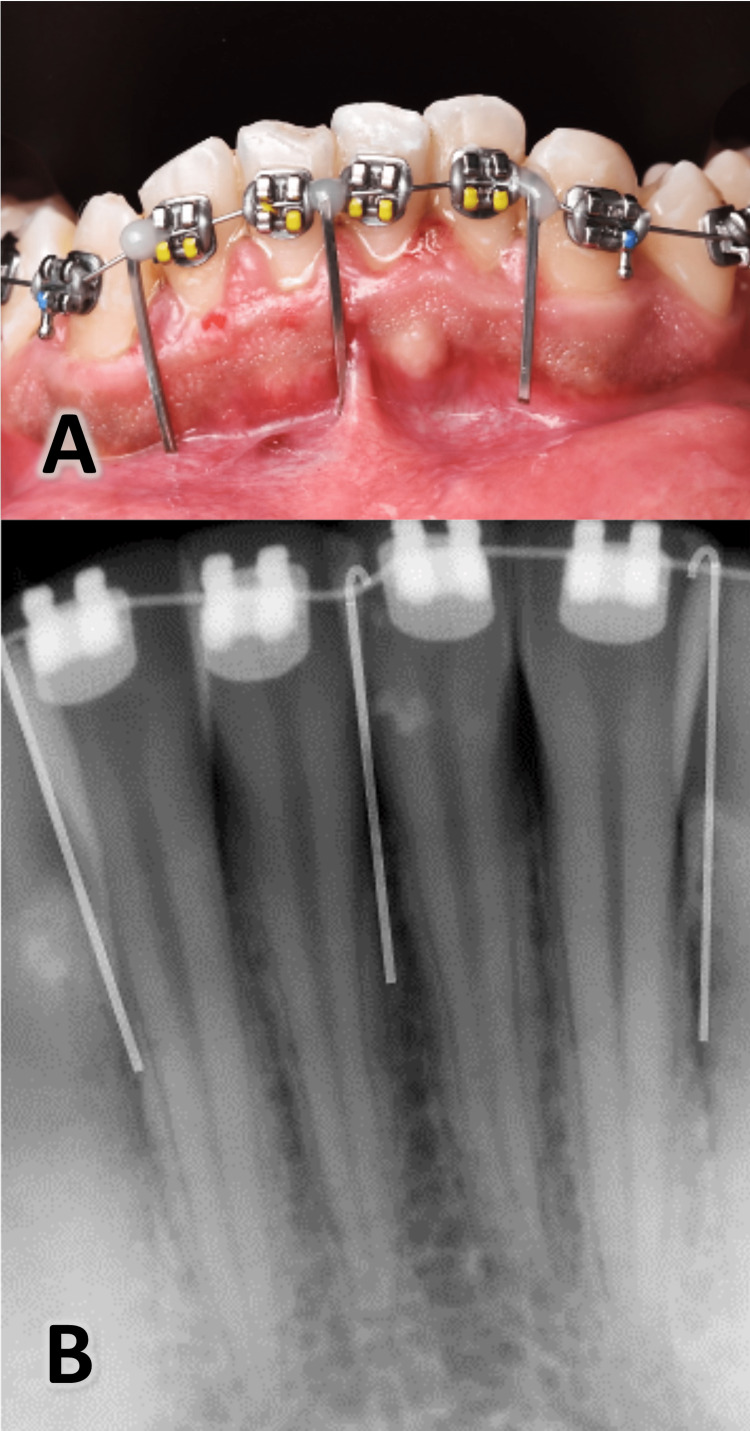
A: Three radiographic metal guides were used before taking digital radiographs. B: The appearance of radiographic metal guides in the taken image to help in determining the best places for the surgical intervention.
